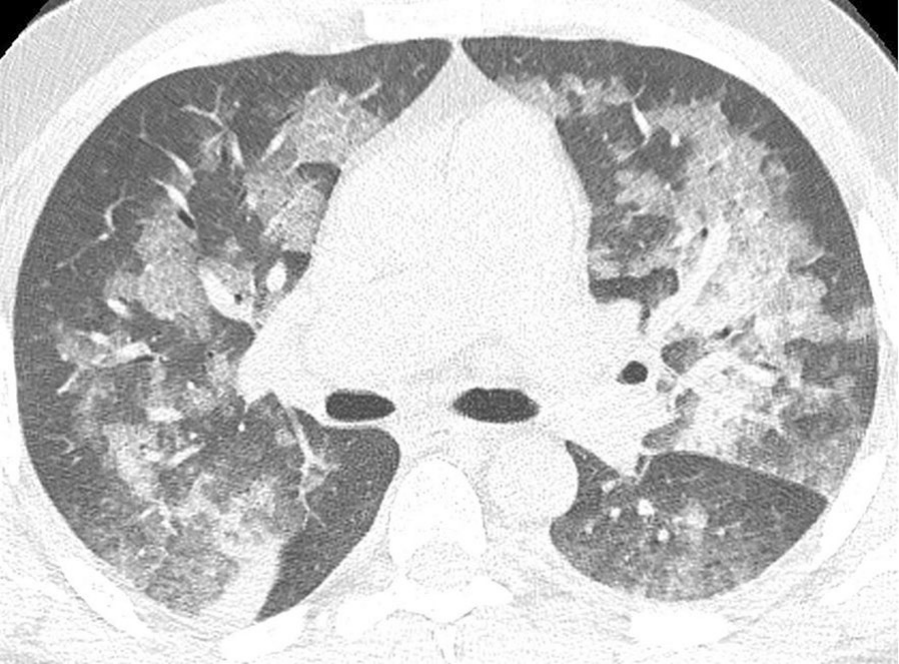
Non-infectious mimickers of indeterminate COVID-19. Pulmonary alveolar proteinosis proved by bronchoalveolar lavage (BAL) in a 38-year-old male smoker, axial CT image shows asymmetric bilateral patchy areas of GGO superimposed with interlobular septal thickening and crazy paving pattern, right-side fissural effusion is also noted
